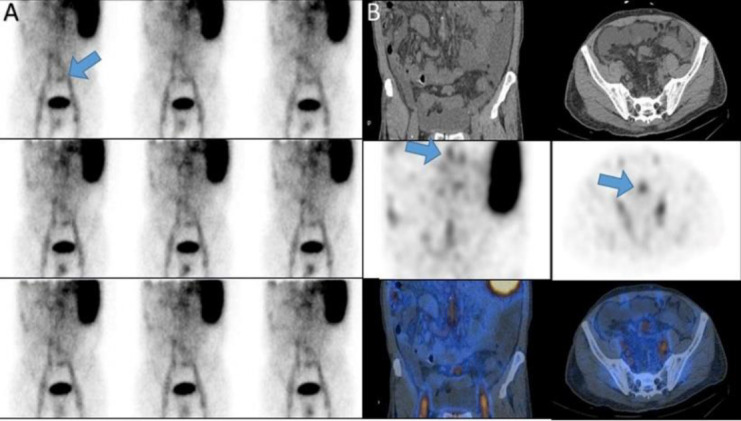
Varicose vessels in a cirrhotic patient on planar (A) and SPECT/CT (B)
